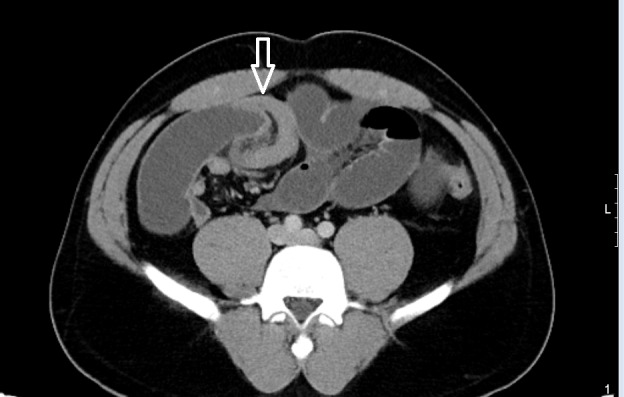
Computed tomography of abdomen - coronal plane
